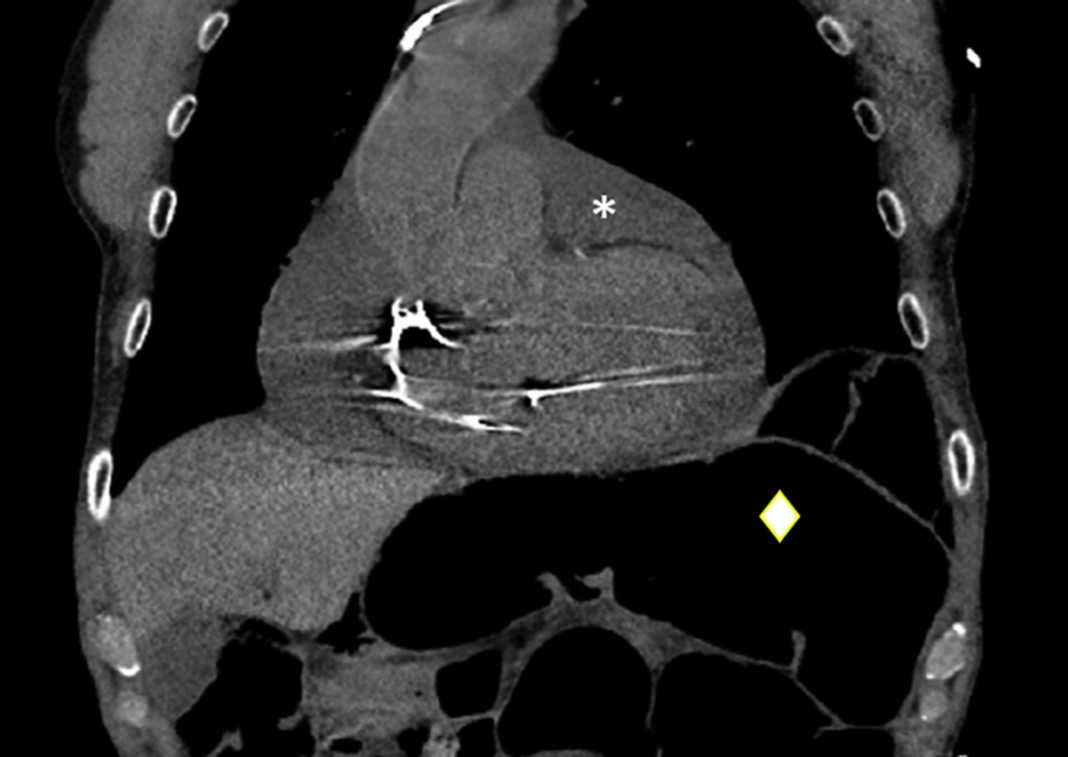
CT Imaging Computed tomography (CT) scan showing evidence of a large pericardial effusion (asterisk) and a distended colon (diamond).
